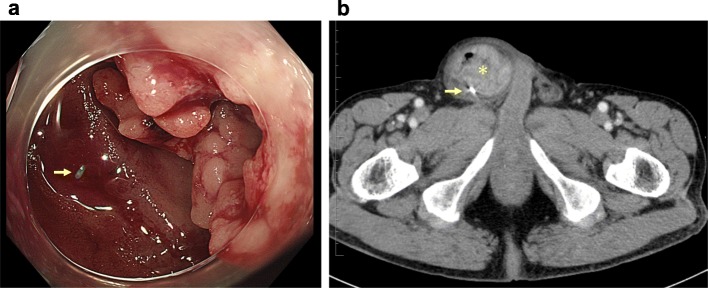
a Colonoscopy revealed bleeding through the ileocecal valve. Therefore, we advanced the scope into the ileum and found an annular stricture by a tumor, for which endoscopic biopsy and clip-marking (arrow) of the tumor were performed. b Enhanced CT demonstrated a hypervascular solid tumor (asterisk) and a metal clip (arrow) by colonoscopy in the right inguinal hernia
